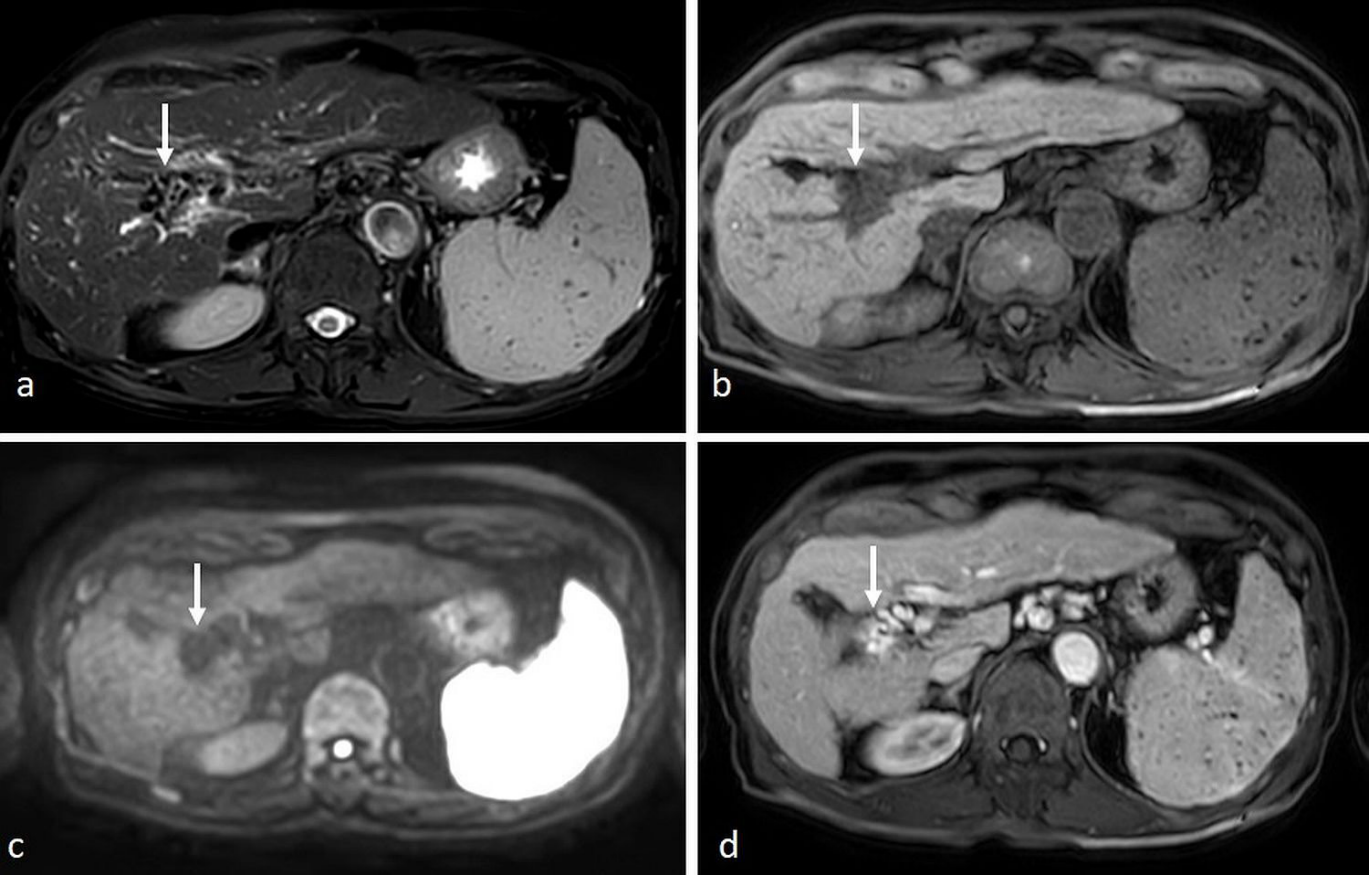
A 74-year-old gentleman with cirrhosis underwent MRI with Primovist for evaluation of a small hepatic lesion on surveillance ultrasound. (a) On axial *T*
